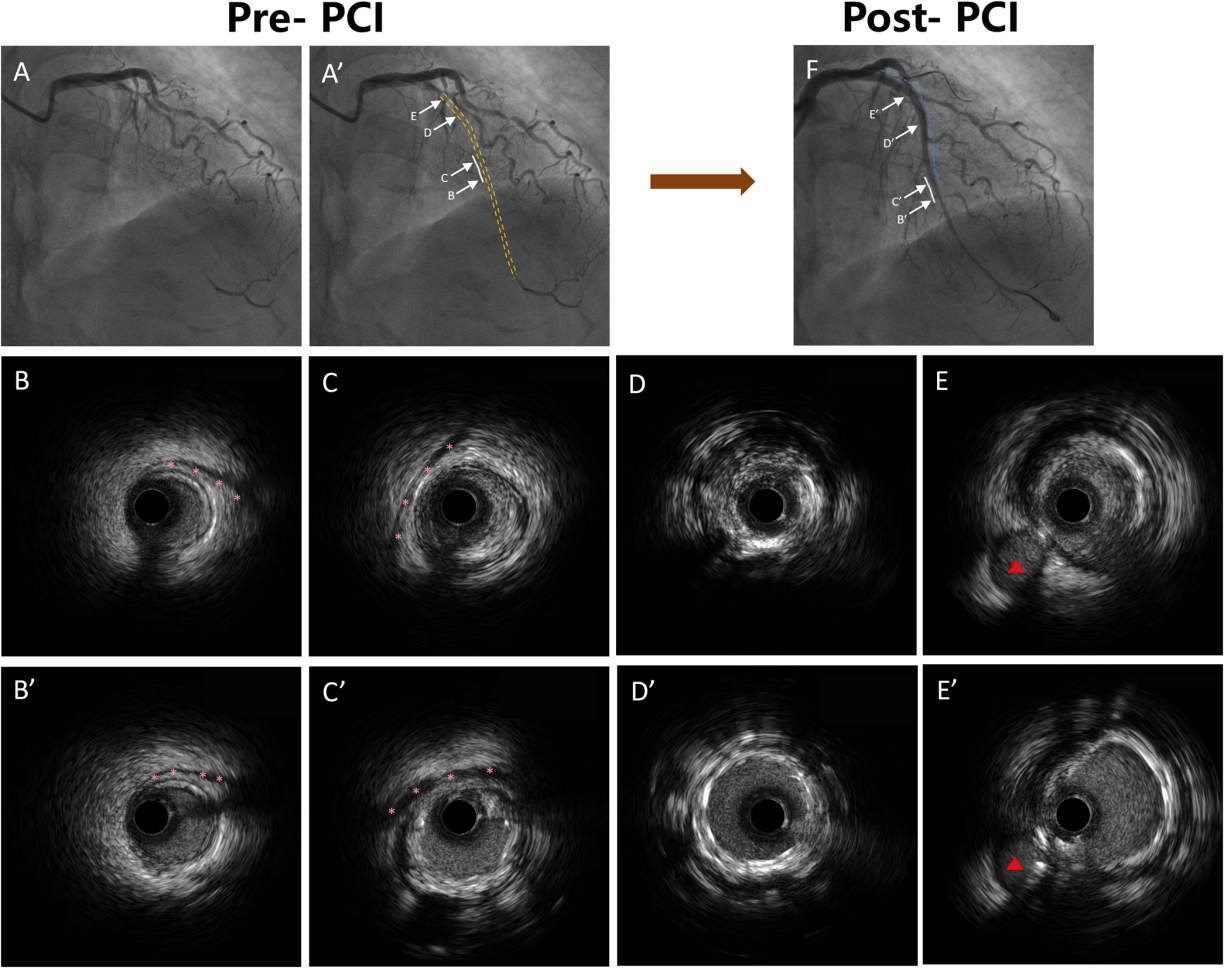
Representative coronary angiography and IVUS images demonstrating MB morphology and stent implantation strategy. (A) A’: Coronary angiography demonstrating the LAD with MB segments marked. The white solid lines indicate MB segments, and the yellow dashed line indicates the occluded segment identified by angiography. (B) B’: IVUS image showing the distal segment of the MB without any plaque formation. The pink asterisks indicate the stent edges. (C) C’: IVUS image showing plaque formation in the proximal segment of the MB, with the stent extending into the bridged segment. The pink asterisks indicate the stent edges. (D) D’: IVUS image showing stent implantation within the CTO segment without MB. (E) E’: IVUS image showing stent implantation in the proximal LAD segment outside of the CTO lesion. The red triangles indicate the diagonal branch. (F) Coronary angiography image showing the LAD after stent implantation. The blue dashed lines indicate the stented segments, and the white solid lines indicate the MB segments. IVUS, intravascular ultrasound; MB, myocardial bridge; LAD, left anterior descending; PCI, percutaneous coronary intervention.
